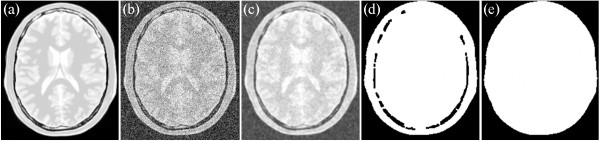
An example of extracting background from PD-weighted MRI. (a) the original PD weight MRI, (b) the MRI with Rician noise, (c) the result of the median filtering, (d) the result of the morphological close operation, and (e) the result of the brain region.
